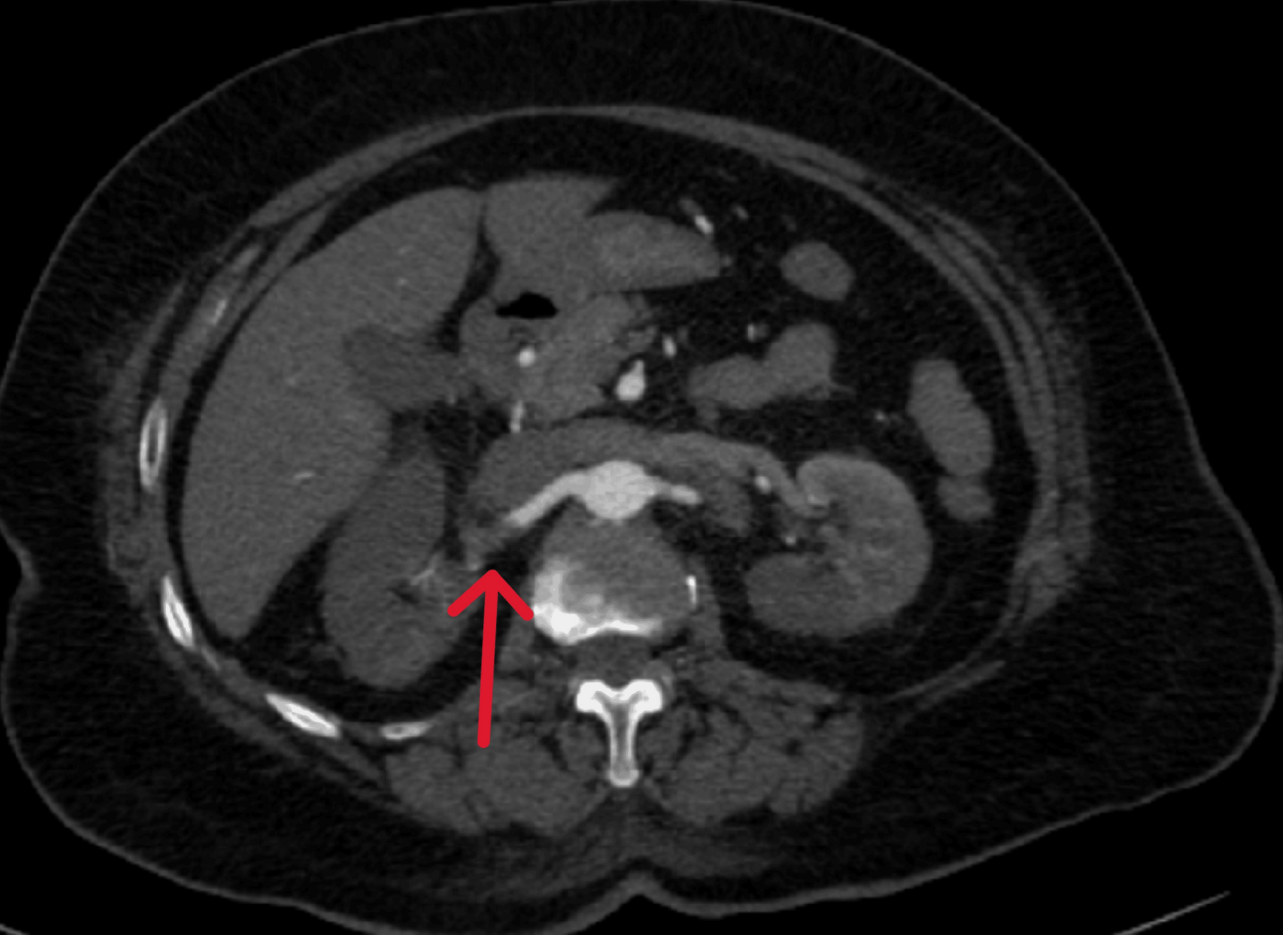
Case 1: Axial CT image showing a filling defect (red arrow) in the right renal artery, consistent with thrombus
